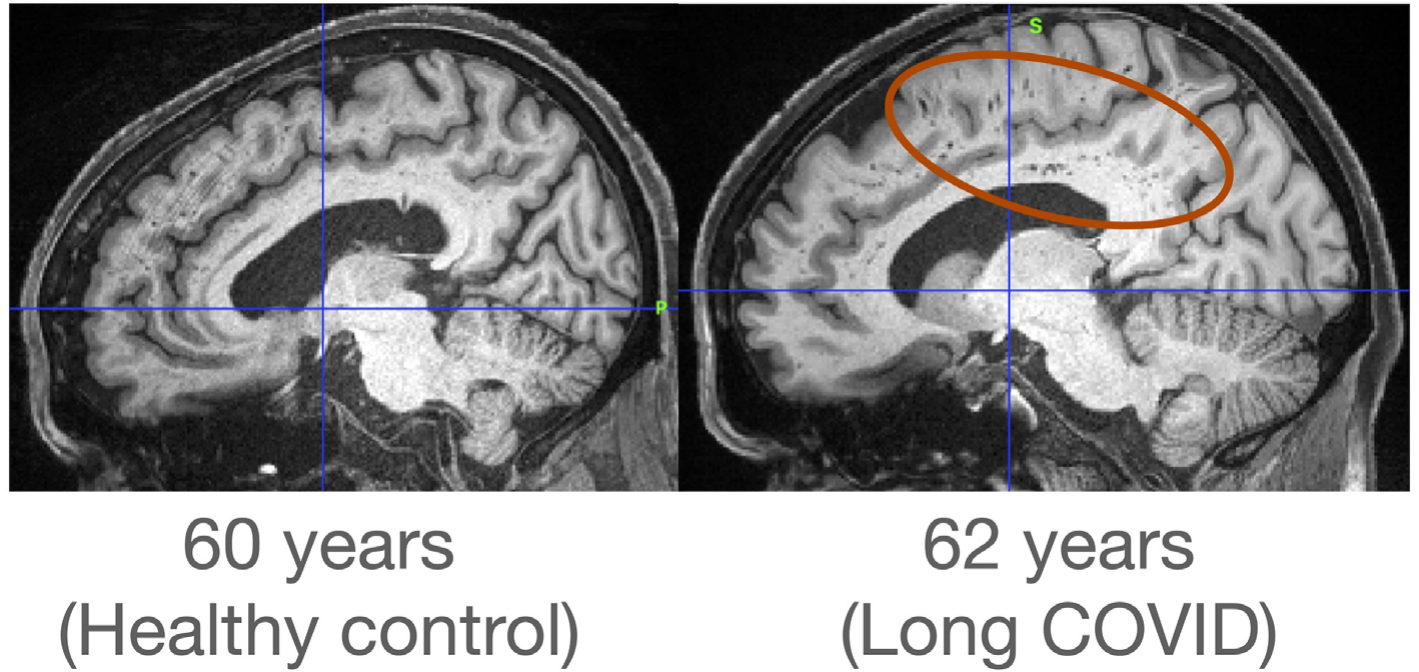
T1‐weighted MR images for a healthy control of age 60 years and a participant diagnosed with Long COVID of age 62 years. The red circle denotes white matter hyperintensities.
